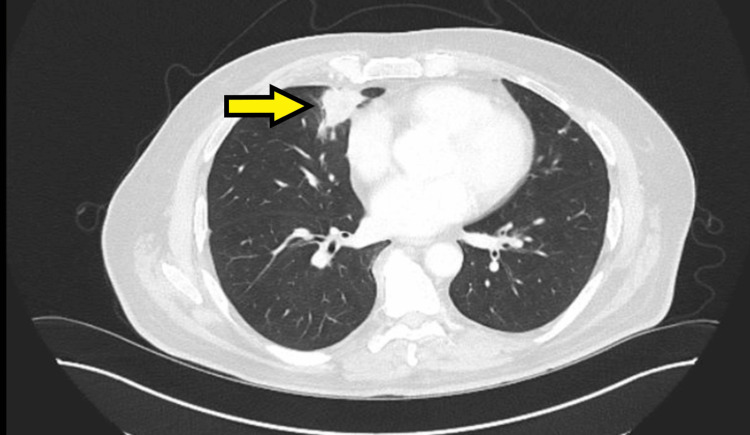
Axial CT scan of the chest demonstrating a 31 × 24 mm soft tissue density lesion in the medial segment of the right middle lobe (yellow arrow) CT: computed tomography
